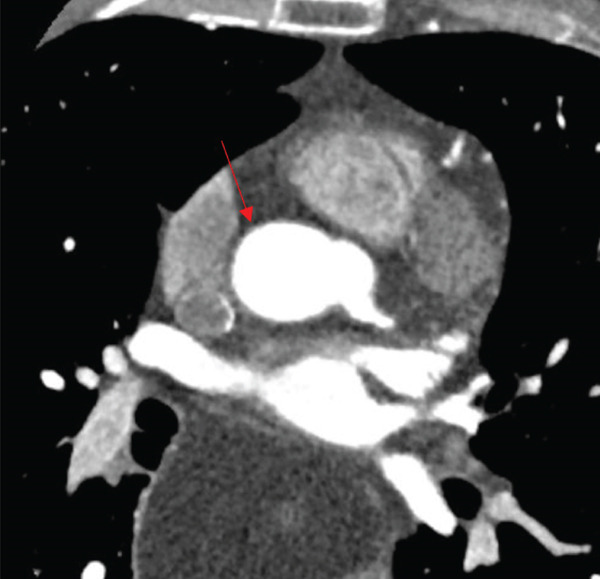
Contrast‐enhanced computed tomography coronary angiography (CTCA) axial view confirming the congenital absence of the right coronary artery ostium (red arrow) from the right coronary sinus.
